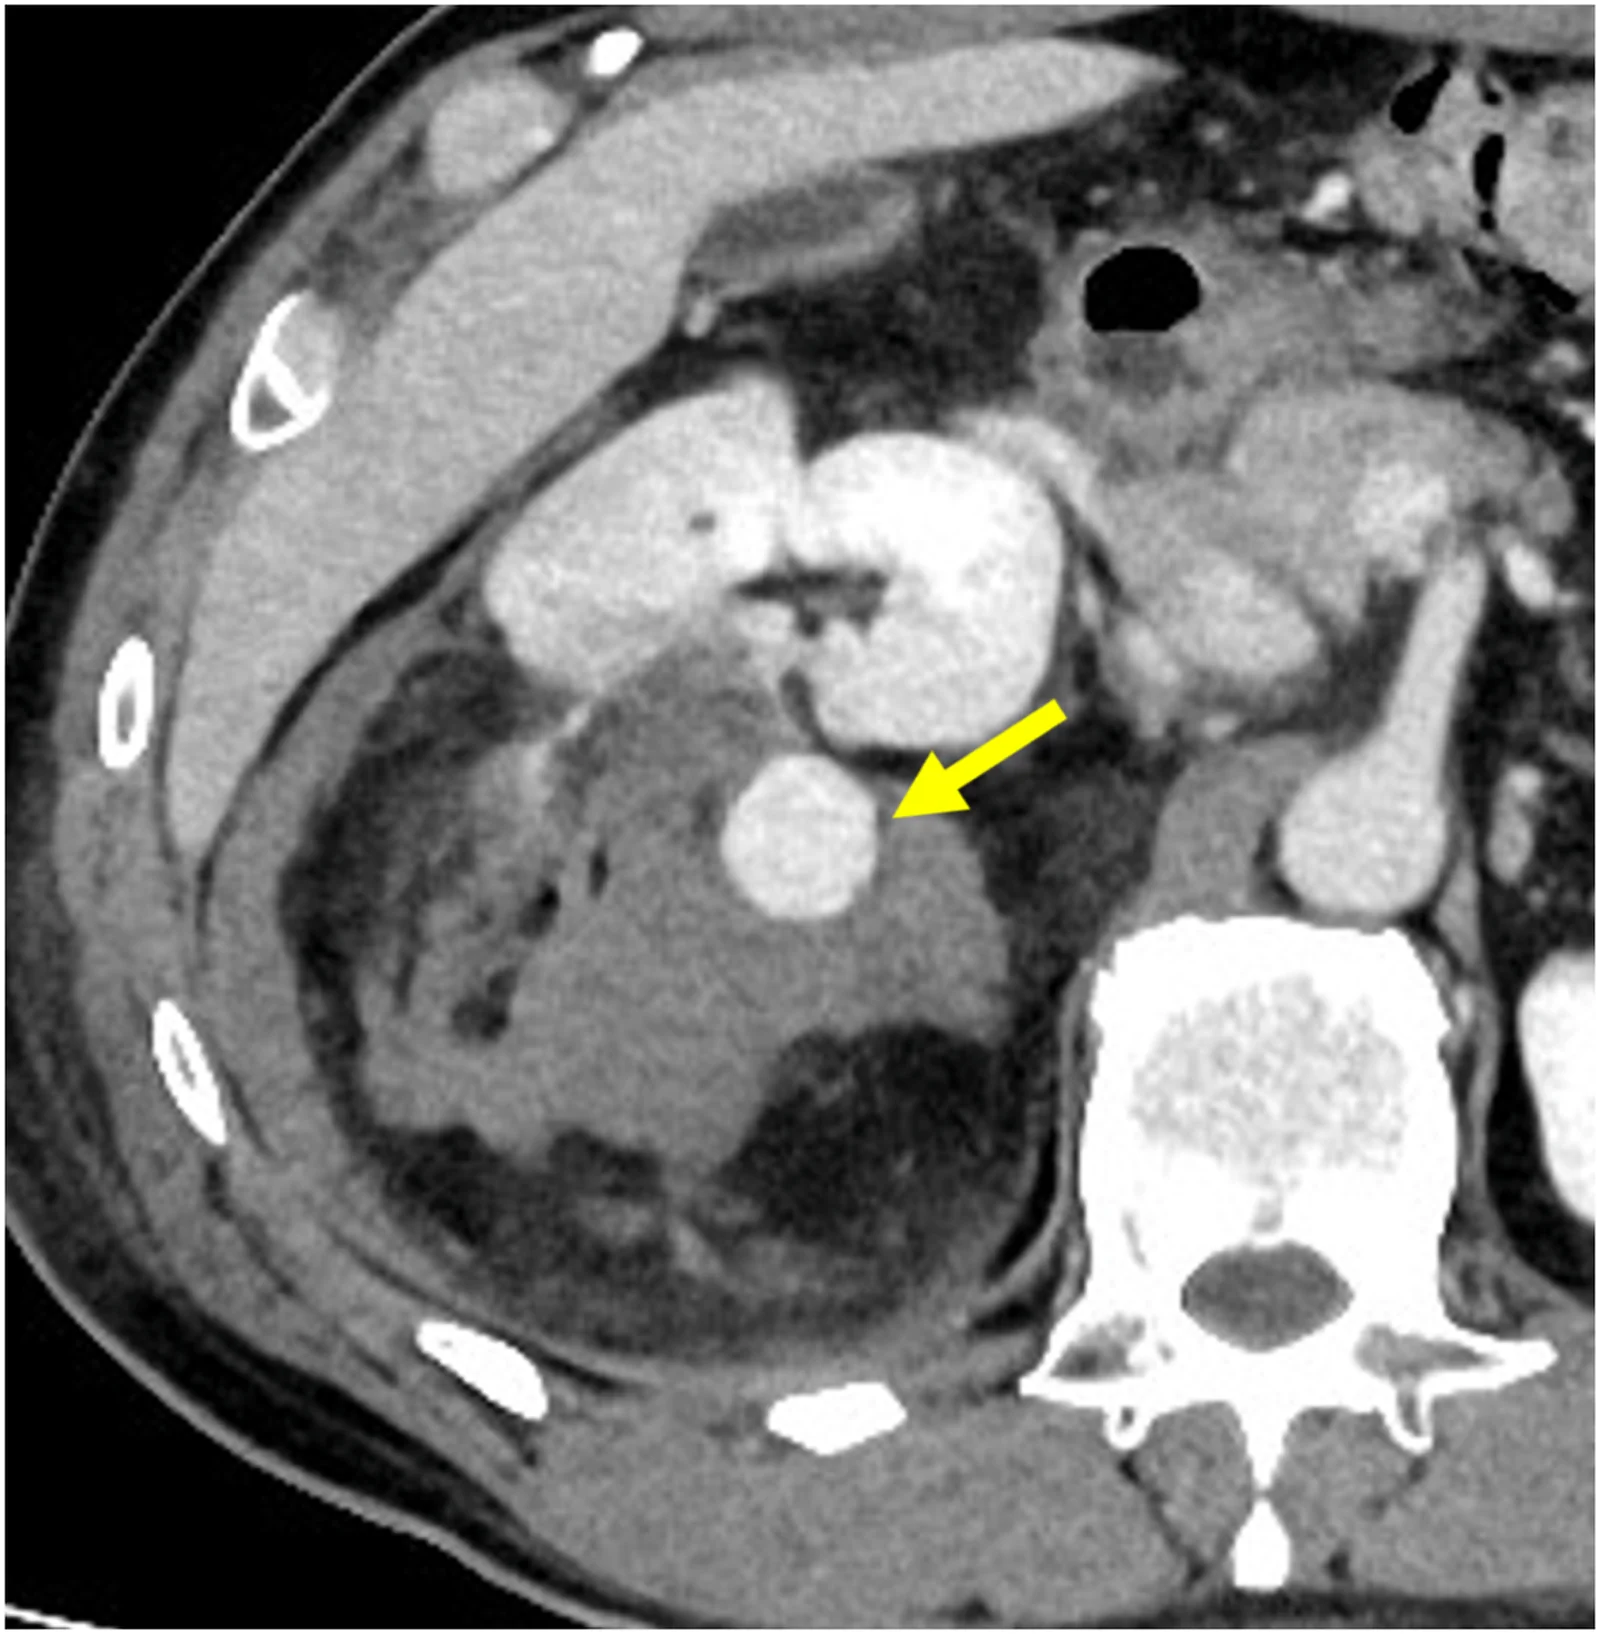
Follow-up contrast-enhanced CT on day 5 after the initial embolization. Axial contrast-enhanced CT image obtained 5 days after the initial embolization demonstrating a newly apparent 20-mm pseudoaneurysm distinct from the lesion identified on the initial CT (arrow).
